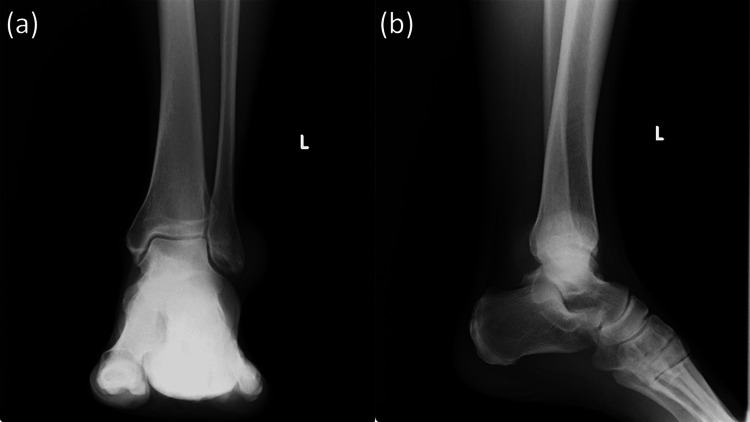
Preoperative ankle radiographs (a) Anteroposterior view and (b) lateral view of the left ankle show preserved joint space without evidence of bone erosion, osteoarthritis, or intra-articular calcification, suggesting the absence of advanced intra-articular involvement.
